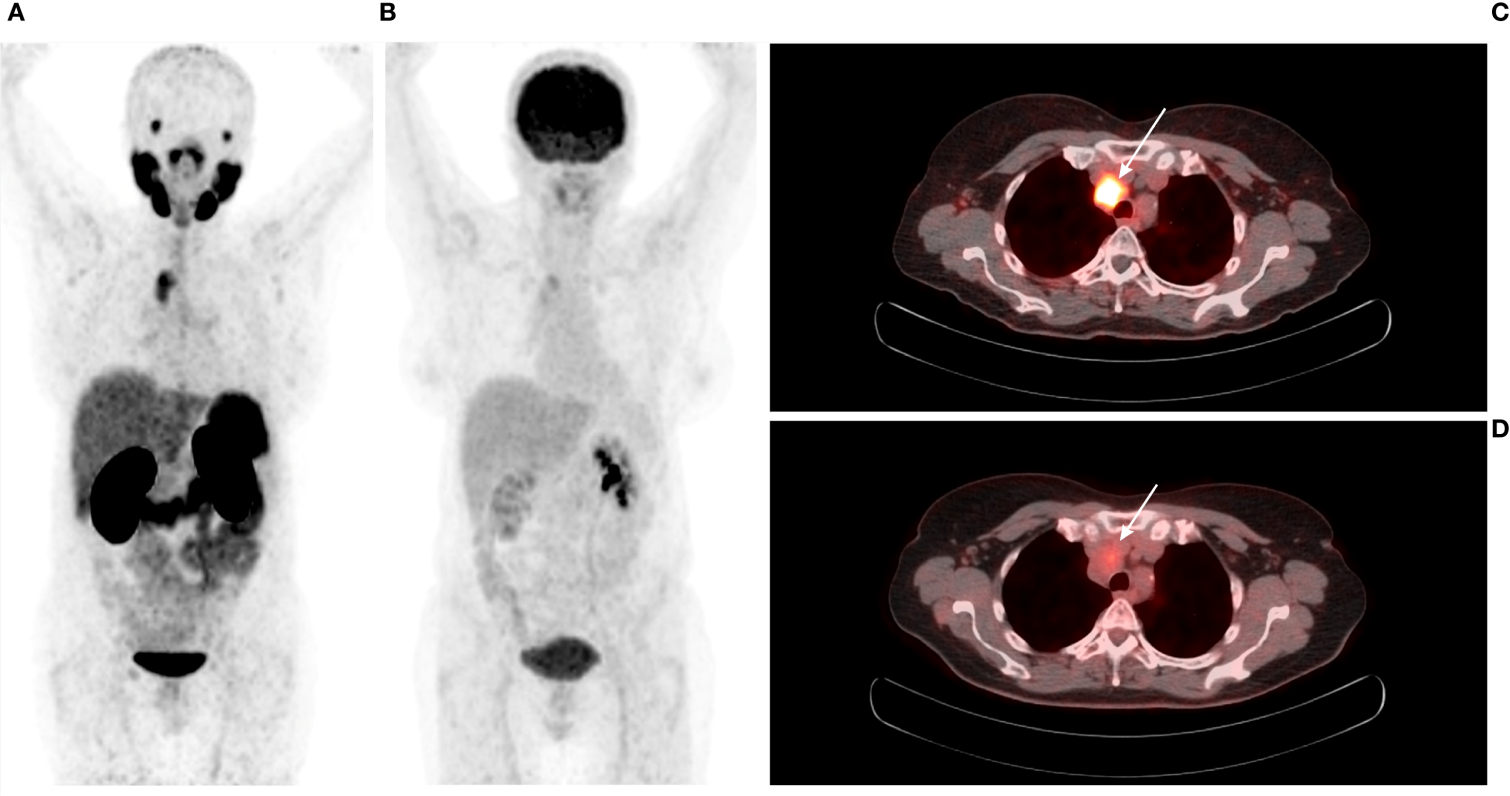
(A) 64-year-old female with medullary thyroid carcinoma and a superior mediastinal mass. (A) [^68^Ga]Ga-PSMA-11 PET MIP. (B) [^18^F]FDG PET MIP. (C) Transaxial view of the fused [^68^Ga]Ga-PSMA-11 PET/CT scan (white arrow). (D) Transaxial view of the fused [^18^F]FDG PET/CT scan (white arrow). The superior mediastinal mass is more avid on [^68^Ga]Ga-PSMA-11 PET (SUV_max_ 7.98; TBR_Blood_ 7.60) than on [^18^F]FDG PET (SUV_max_ 3.81; TBR_Blood_ 1.85).
